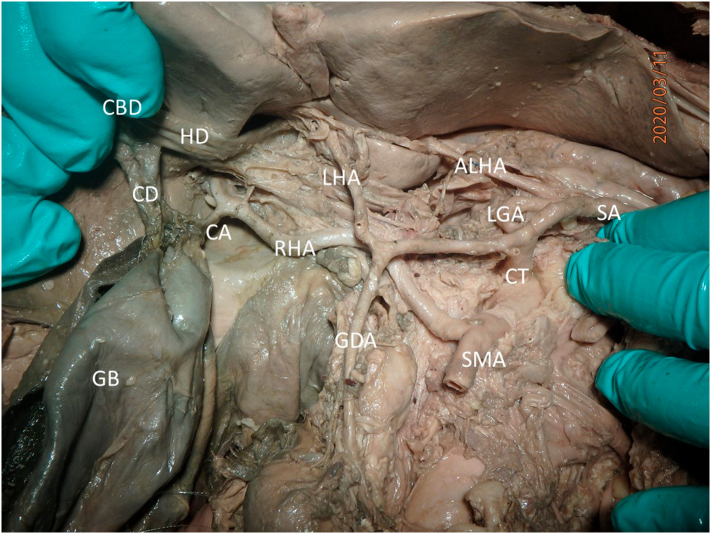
Reflection of the common bile duct (CBD), hepatic duct (HD), and the cystic duct (CD) revealed the right hepatic artery (RHA) as a branch off the superior mesenteric artery (SMA). Additionally, an accessory left hepatic artery (ALHA) was seen branching from the left gastric artery (LGA). CT: celiac trunk; CHA: common hepatic artery; SA: splenic artery; LHA: left hepatic artery; GDA: gastroduodenal artery; GB: gallbladder; CA: cystic artery.
